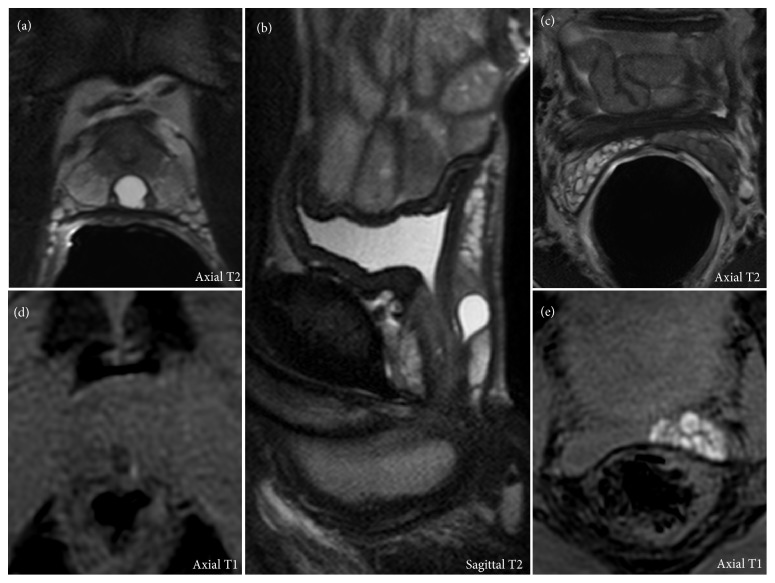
Summary sheet of 3-tesla MRI examination. Panels (a), (b), and (c) are acquired with an endorectal coil. Panels (d) and (e) are acquired without an endorectal coil and are the prebolus phase of the dynamic contrast-enhanced MR perfusion serving as T1-weighted images without gadolinium. Panels (a), (b), and (d) are centered on the cystic lesion. Panels (c) and (e) show stigmata of hematospermia in the left seminal vesicle. The orthogonal planes are specified in each panel.
